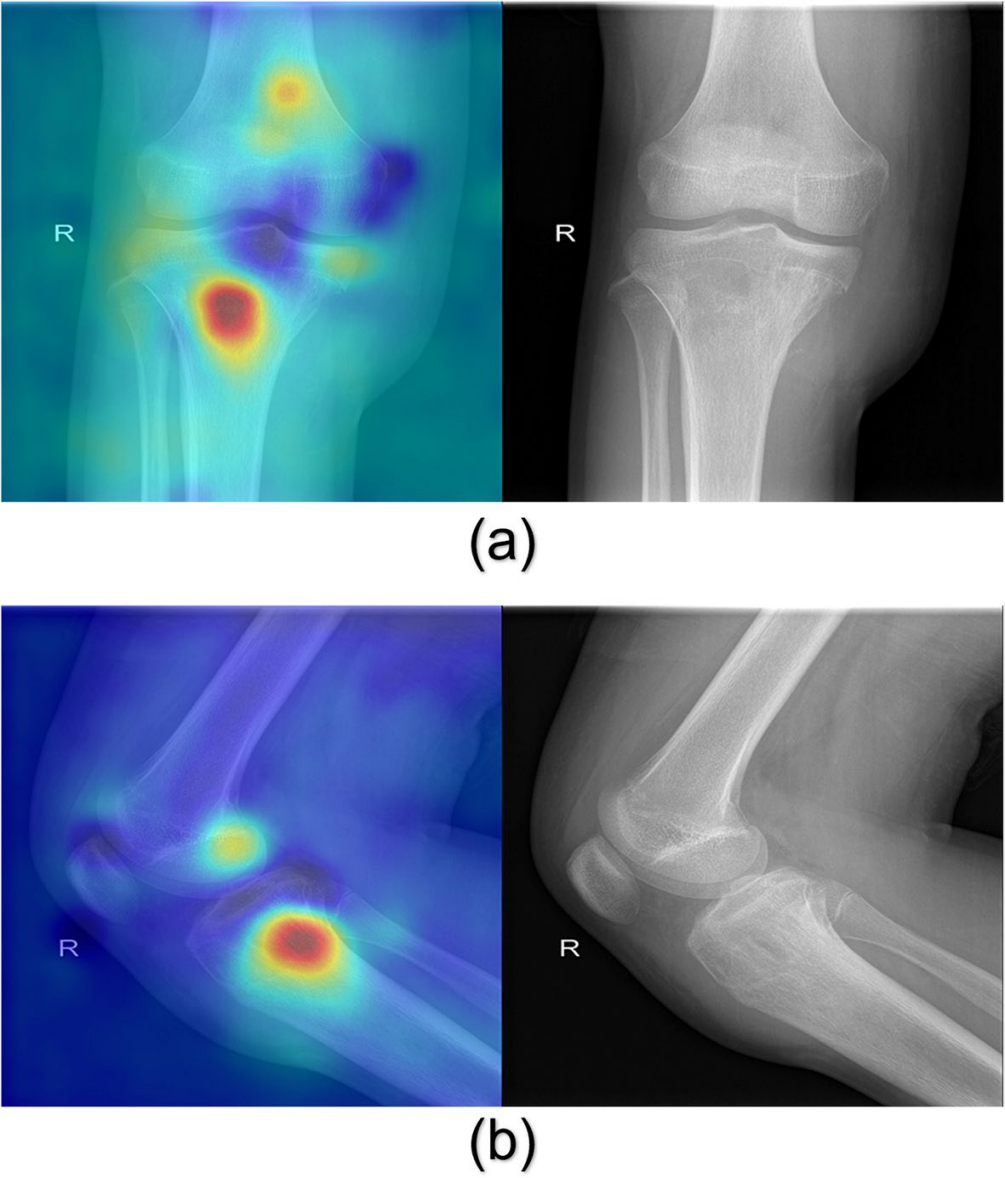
Anteroposterior (a) and lateral (b) radiographs of OS of the proximal tibia and their overlapping Grad-CAM maps
